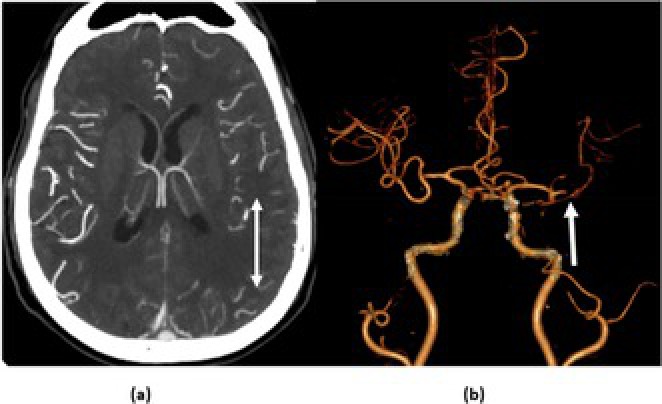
FIGURE 1 Axial CT sections of CT angiogram study (a) and 3D reconstruction of intracranial vessels show paucity of left middle cerebral artery (MCA) territory M3 and M4 cortical branches (double headed arrow) and stenosis at distal left M1 MCA on 3D images (arrow).
